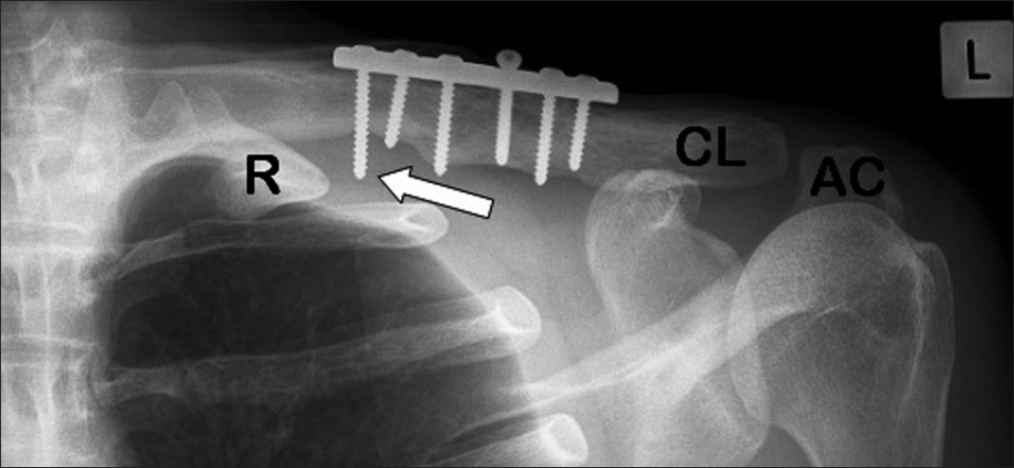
Plain radiograph of left clavicle. (AC: Acromion. CL: Clavicle. R: 1^st^ rib). Arrow depicts prominent medial screw lateral to the 1^st^ rib
